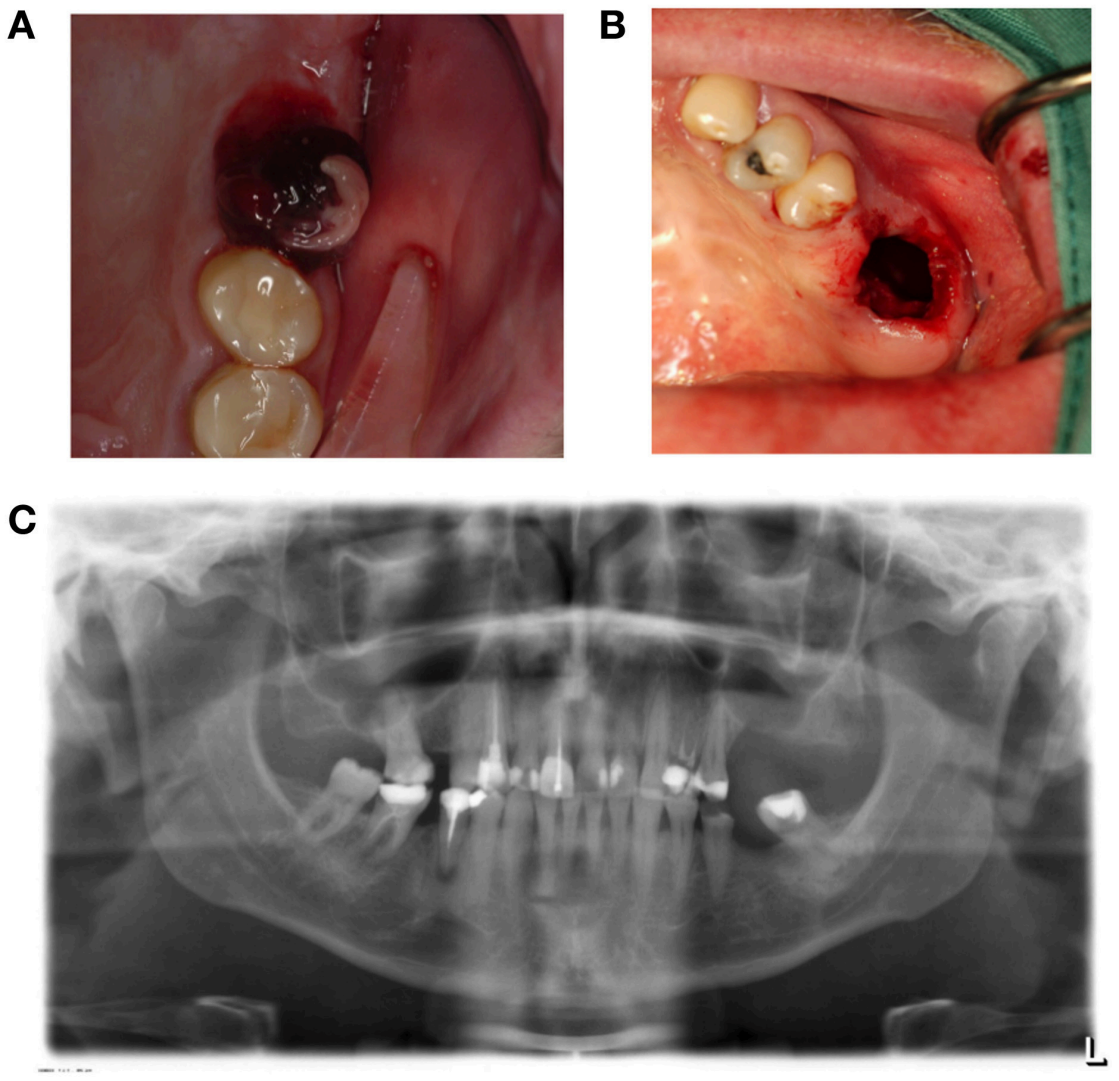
(A) A sinus polyp is a manifestation of a poorly healing antrum perforation that does not spontaneously close. This can lead to the bony height of the rest alveolus being too small following tooth extraction, and the connection between the mouth and antrum being too wide. (B) Panoramic radiography shows loss of bone height separating the antrum of the oral cavity in region 26. (C) Brightening of the wound edges reveals the dimension of the connection between the antrum and oral cavity.
